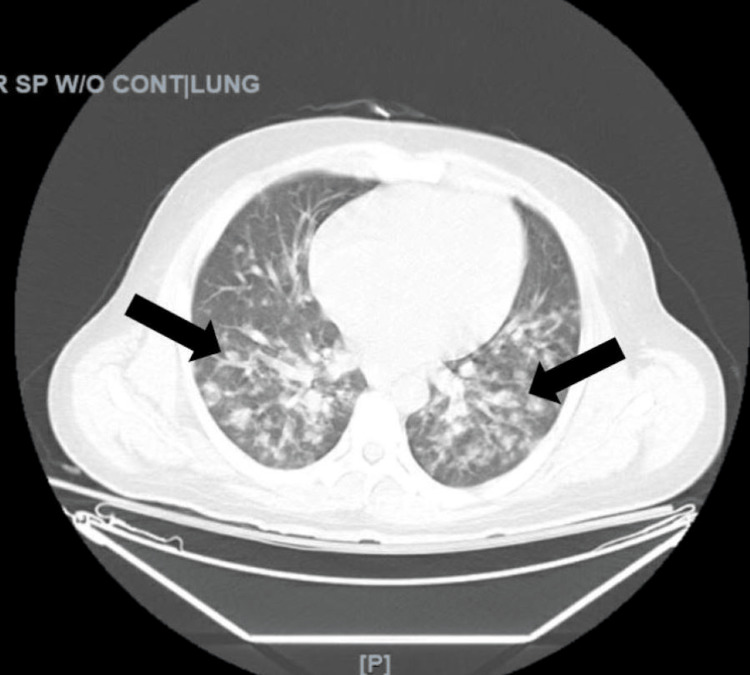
CT of the chest showing nodular infiltrates in both lung fields, suggestive of septic emboli.
